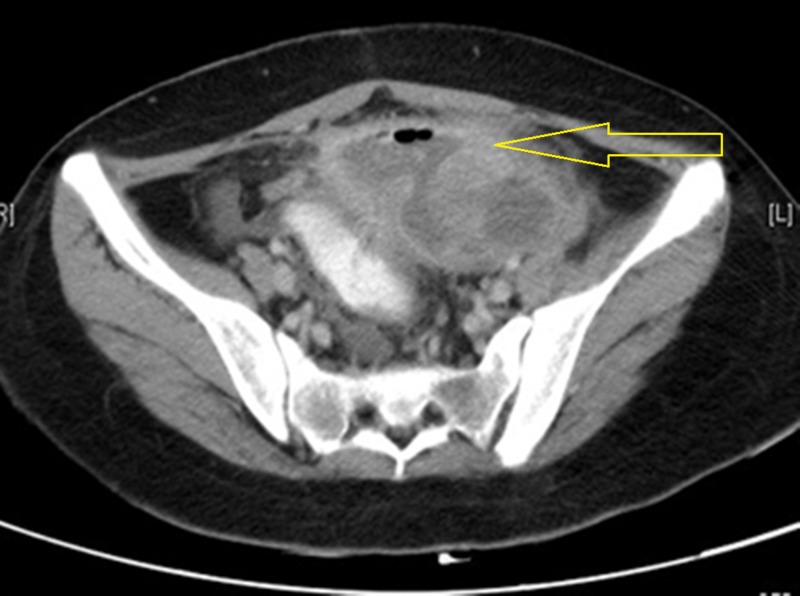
Contrast-enhanced computed tomography (CECT) of the abdomen and pelvis showing multiloculated pelvic collection with significant sigmoid wall thickening indicating sigmoid perforation
